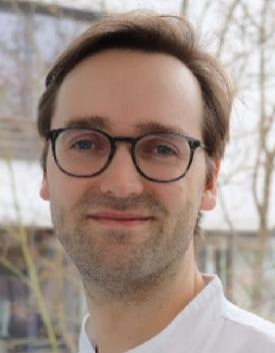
Dr Sören J. Backhaus is a consultant cardiologist at Kerckhoff Heart Centre Bad Nauheim and senior lecturer at Justus-Liebig-University Giessen. He completed his cardiology training at University Medical Center Göttingen, where he became consultant cardiologist and senior lecturer in Internal Medicine and Cardiology prior to the acceptance of his role in Bad Nauheim. He specializes in multimodality cardiovascular imaging with particular interest in cardiovascular magnetic resonance imaging in heart failure.
